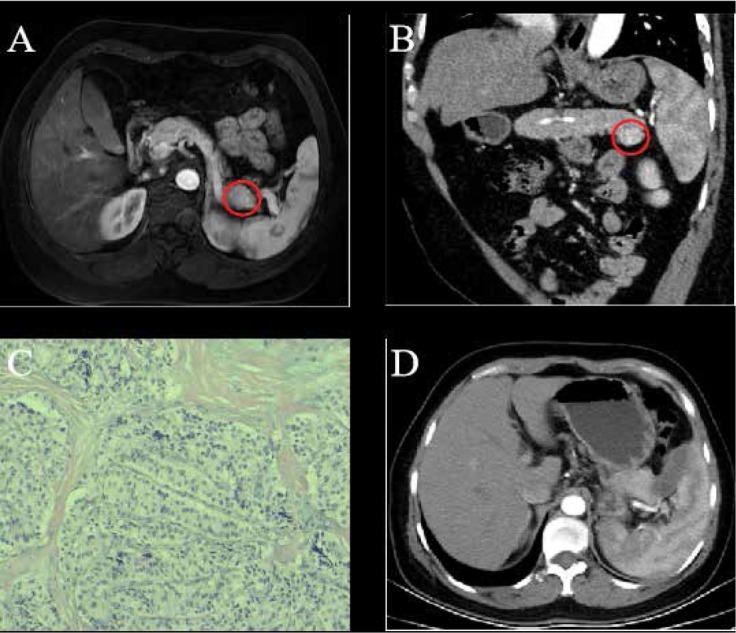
A 44-year-old female with pathology-proven pancreatic insulinoma was treated with laparoscopic RFA. (A) Contrast-enhanced MRI showed a round-like, well-defined lesion in the pancreatic tail in the arterial phase (red cycle). (B) Coronal reconstructed CT image showed the tumor grew in an exophytic pattern (red cycle). (C) Pathology confirmed the diagnosis of pancreatic insulinoma (HE×100). (D) Contrast-enhanced CT showed no residual tumor foci and recurrent lesion with a small volume of fluid collection near the pancreatic tail.
